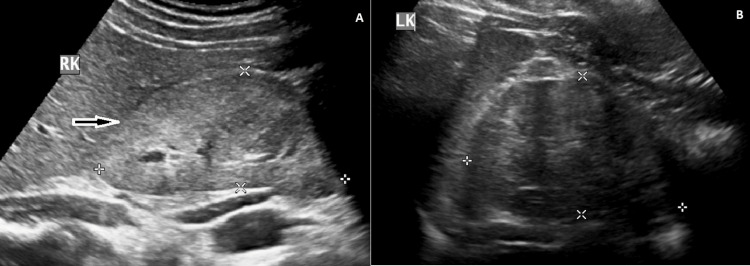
A: ultrasound showing right kidney measuring 8.8 x 4.1 cm with increased echogenicity and lost CMD (white-bordered black arrow (magnification: 600 DPI)) and B: ultrasound showing contracted left kidney measuring 8.5 x 5.3 cm with lost CMD (white-bordered black arrow (magnification: 600 DPI)) CMD: corticomedullary distinction; DPI: dots per inch
